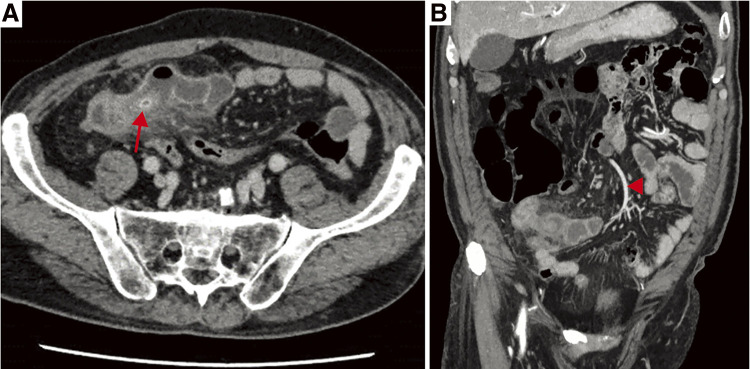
Preoperative contrast-enhancement CT images (Case 2). Axial (A) and coronal (B) views showed a duplicated intestinal tract communicating to the small intestine fed by ileocolic artery (red arrowhead). There was a high-density fecal stone detected in the root area (red arrow).
